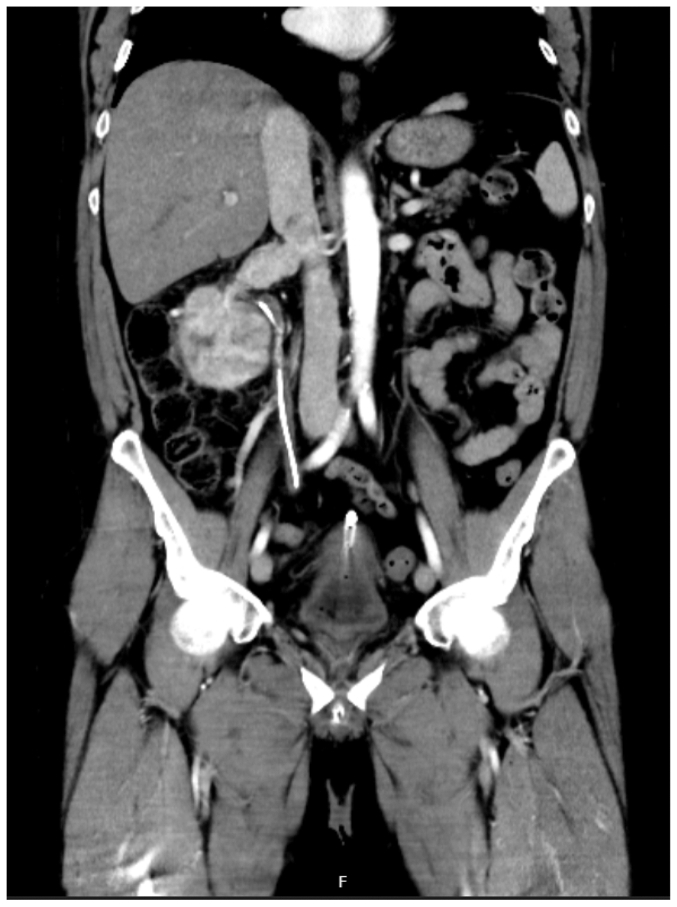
A computed tomography (*CT*) intravenous pyelogram demonstrating a distended and occluded right renal vein, and filling defects evident with the pararenal inferior vena cava (*IVC*) extending to the level of the caudate lobe.
